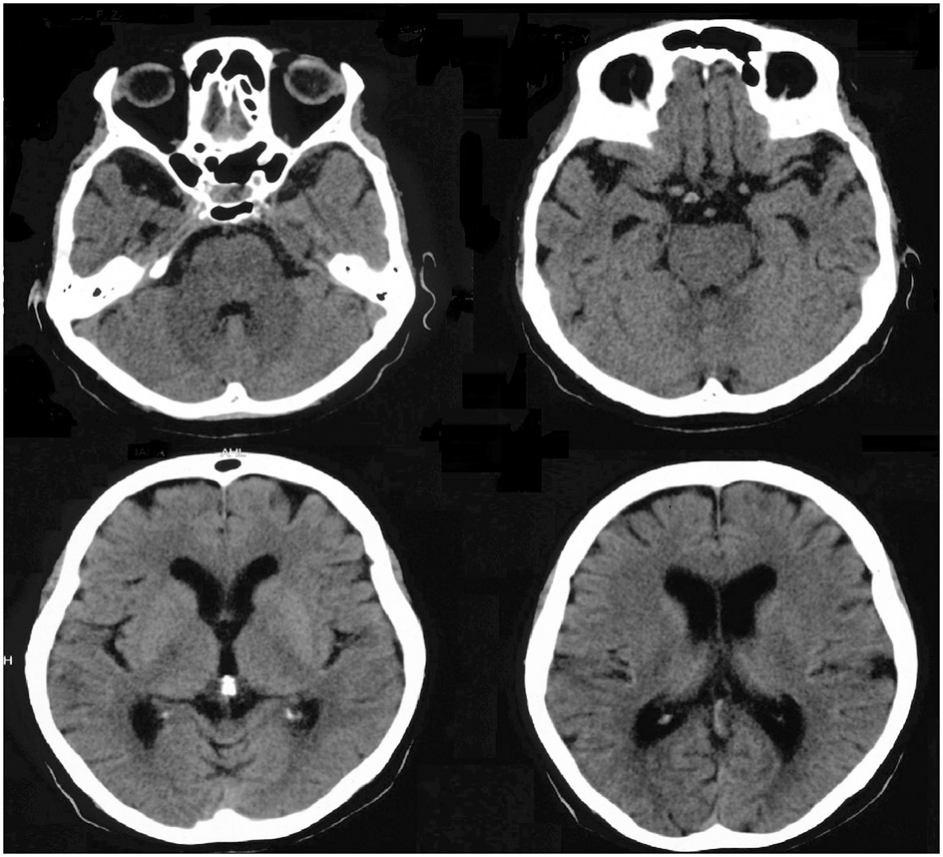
Brain computed tomography scan performed in 2009 showing marked atrophy in bilateral temporopolar and frontal regions.
